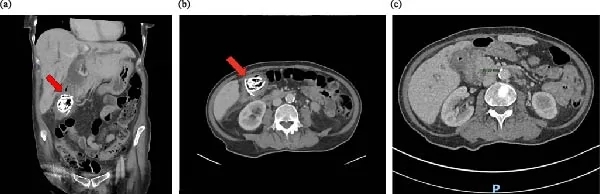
Contrast‐enhanced CT images demonstrating the cholecystocolonic fistula and associated choledocholithiasis. (a) Coronal and (b) axial images show a large peripherally calcified gallstone within the hepatic flexure, immediately adjacent to the collapsed, thick‐walled gallbladder fundus, with surrounding inflammatory change and intraluminal gas. (c) Axial image shows an ~11 mm calculus impacted in the distal common bile duct (calipers), with upstream biliary dilatation.
